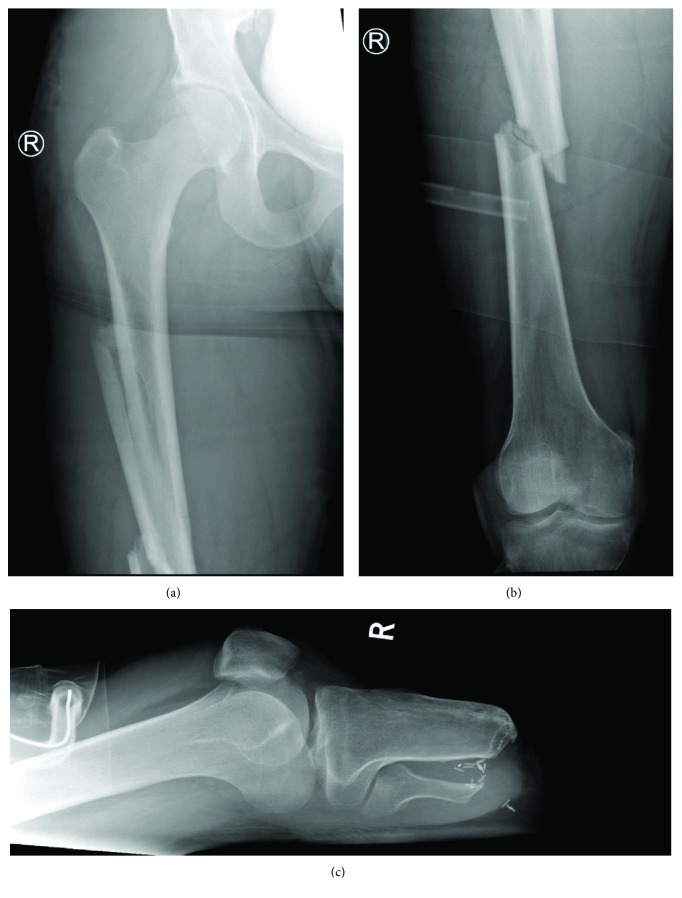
Standard anteroposterior radiograph of the right hip and femur (a), anteroposterior radiograph of the distal right femur (b), and standard lateral radiograph of the right knee (c).
